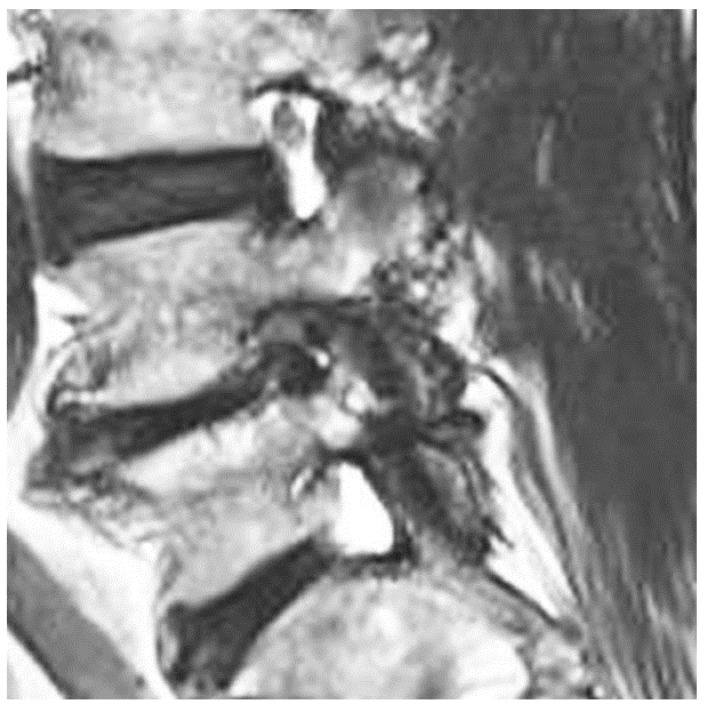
Mid right foraminal MRI of lumbar spine. It showed severe grade 3 Lee et al. foraminal stenosis with morphological change of right L4 exiting nerve root in right L4/5 foramen.
